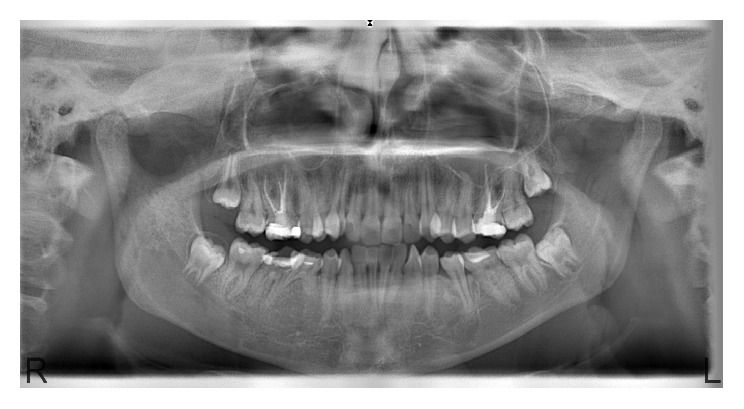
Panoramic radiography shows ground-glass ill-defined lesions in the three different regions of the maxilla and mandible.
